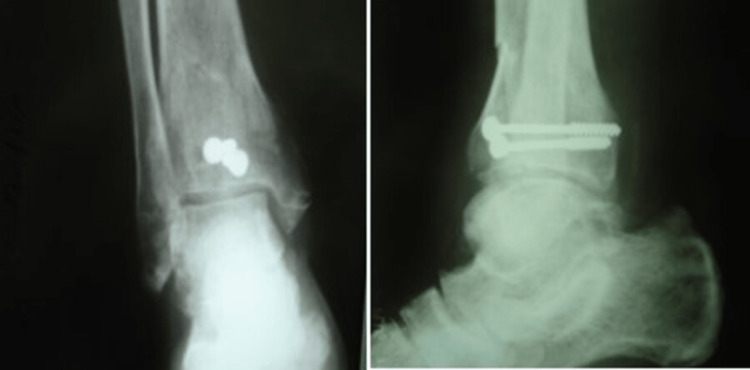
Anteroposterior and lateral postoperative (12 weeks) X-ray
